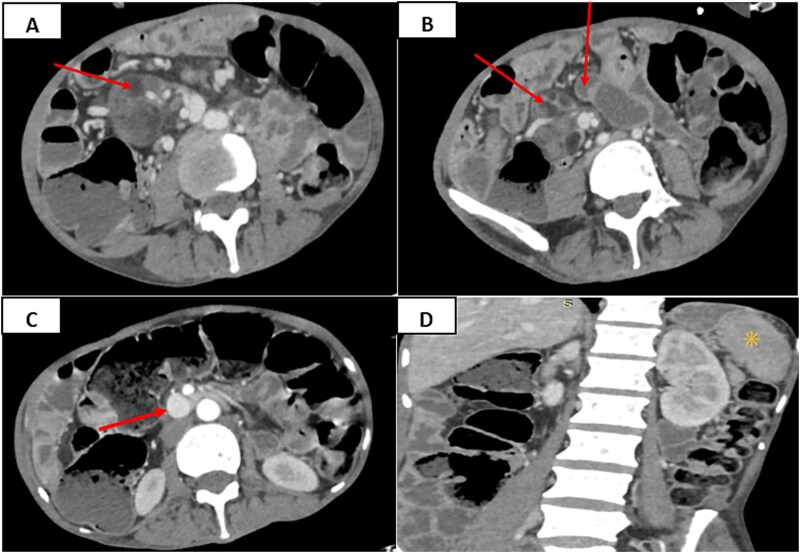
(A, B) axial contrast-enhanced abdominal CT images show conglomerate mesenteric lymph nodes with fat-fluid levels and pseudocystic centers (arrowheads). (C) Axial contrast-enhanced view demonstrates dilation of the superior mesenteric vein, reflecting venous congestion secondary to lymphadenopathic compression (arrowhead). (D) Coronal contrast-enhanced CT image reveals splenic atrophy (asterisk).
